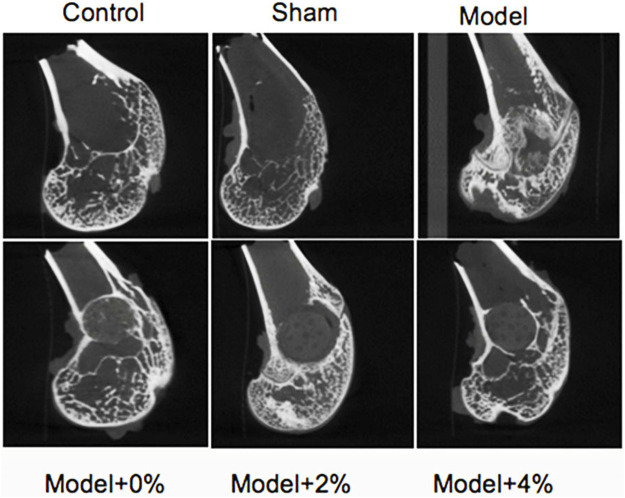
New bone density after 12 weeks as analyzed by micro CT.
